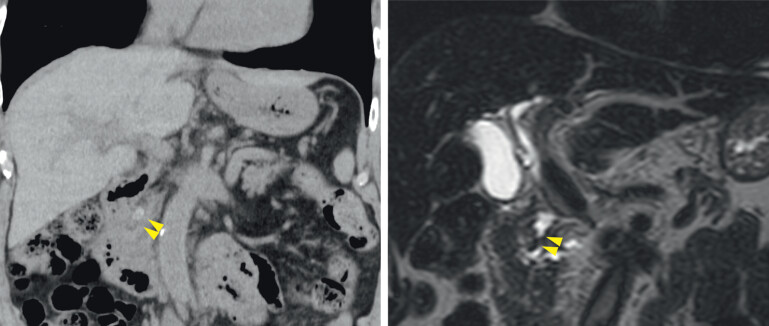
Computed tomographic and magnetic resonance
